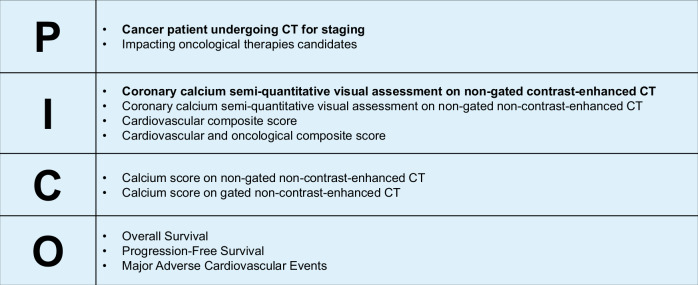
Fig. 5 A theoretical PICO (population, intervention, comparison, outcome) framework addressing the role of semi-quantitative visual assessment of coronary calcium on non-gated contrast-enhanced CT scans in cancer patients
